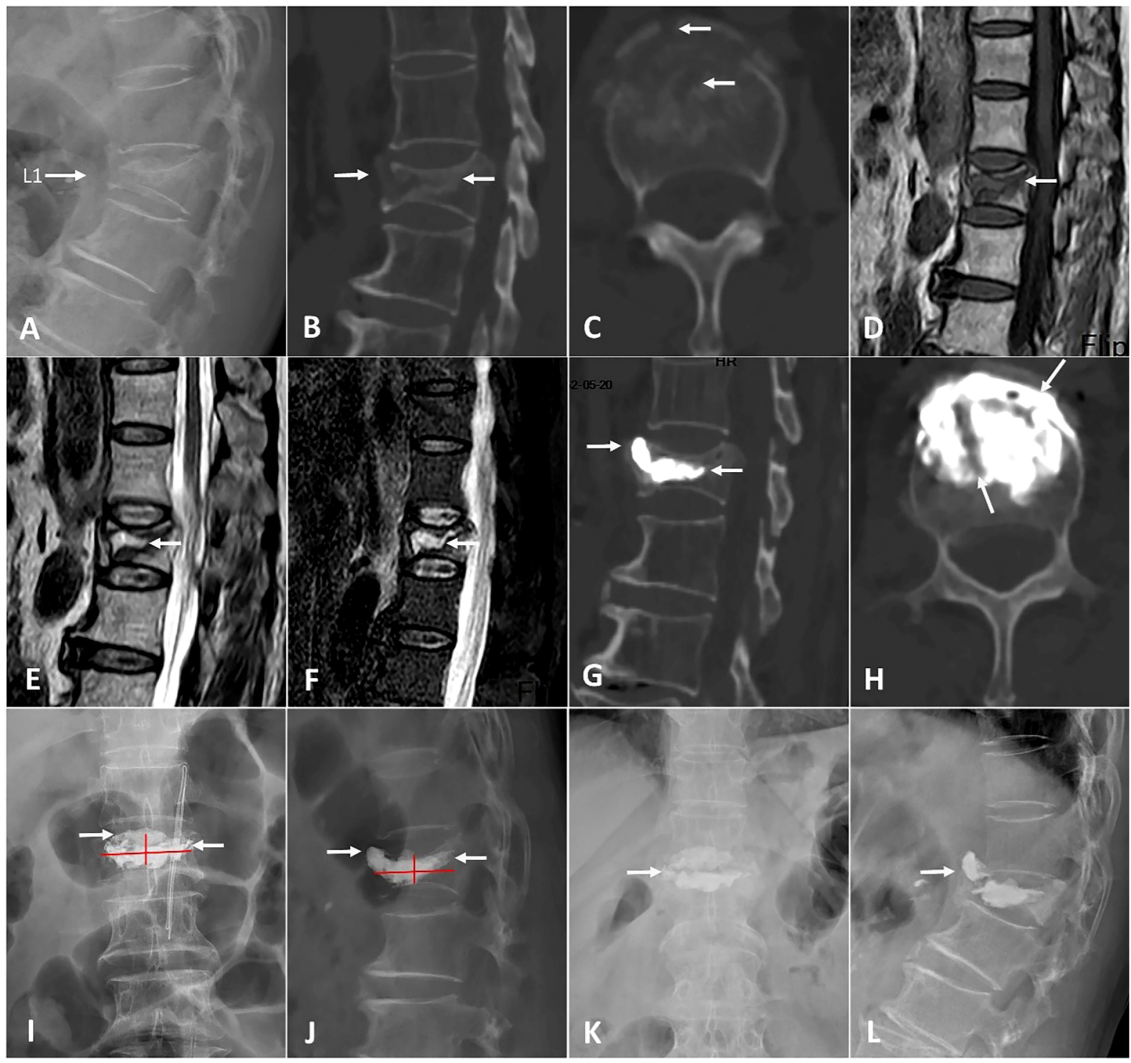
A 70-year-old woman with KD at L1 was operated by T-PKP. (A-C) Lateral X-ray and CT showing an anterior cortex defect and IVC (white arrows). (D) The sagittal T1-weighted MRI exhibiting a low signal intensity in IVC. (E, F) The sagittal T2-weighted and STIR sequences MRI exhibiting a high signal with a surrounding low intensity (double-line sign; DLS). (G-J) Postoperative CT and X-ray displaying a blocky cement pattern. Cements are separated by a fibrocartilaginous membrane in picture (H) (white arrows). (K, L) Anteroposterior and lateral X-ray at a final follow-up, showing vertebral collapse [white arrow in picture (K)] and bone cement displacement [white arrow in picture (L)].
